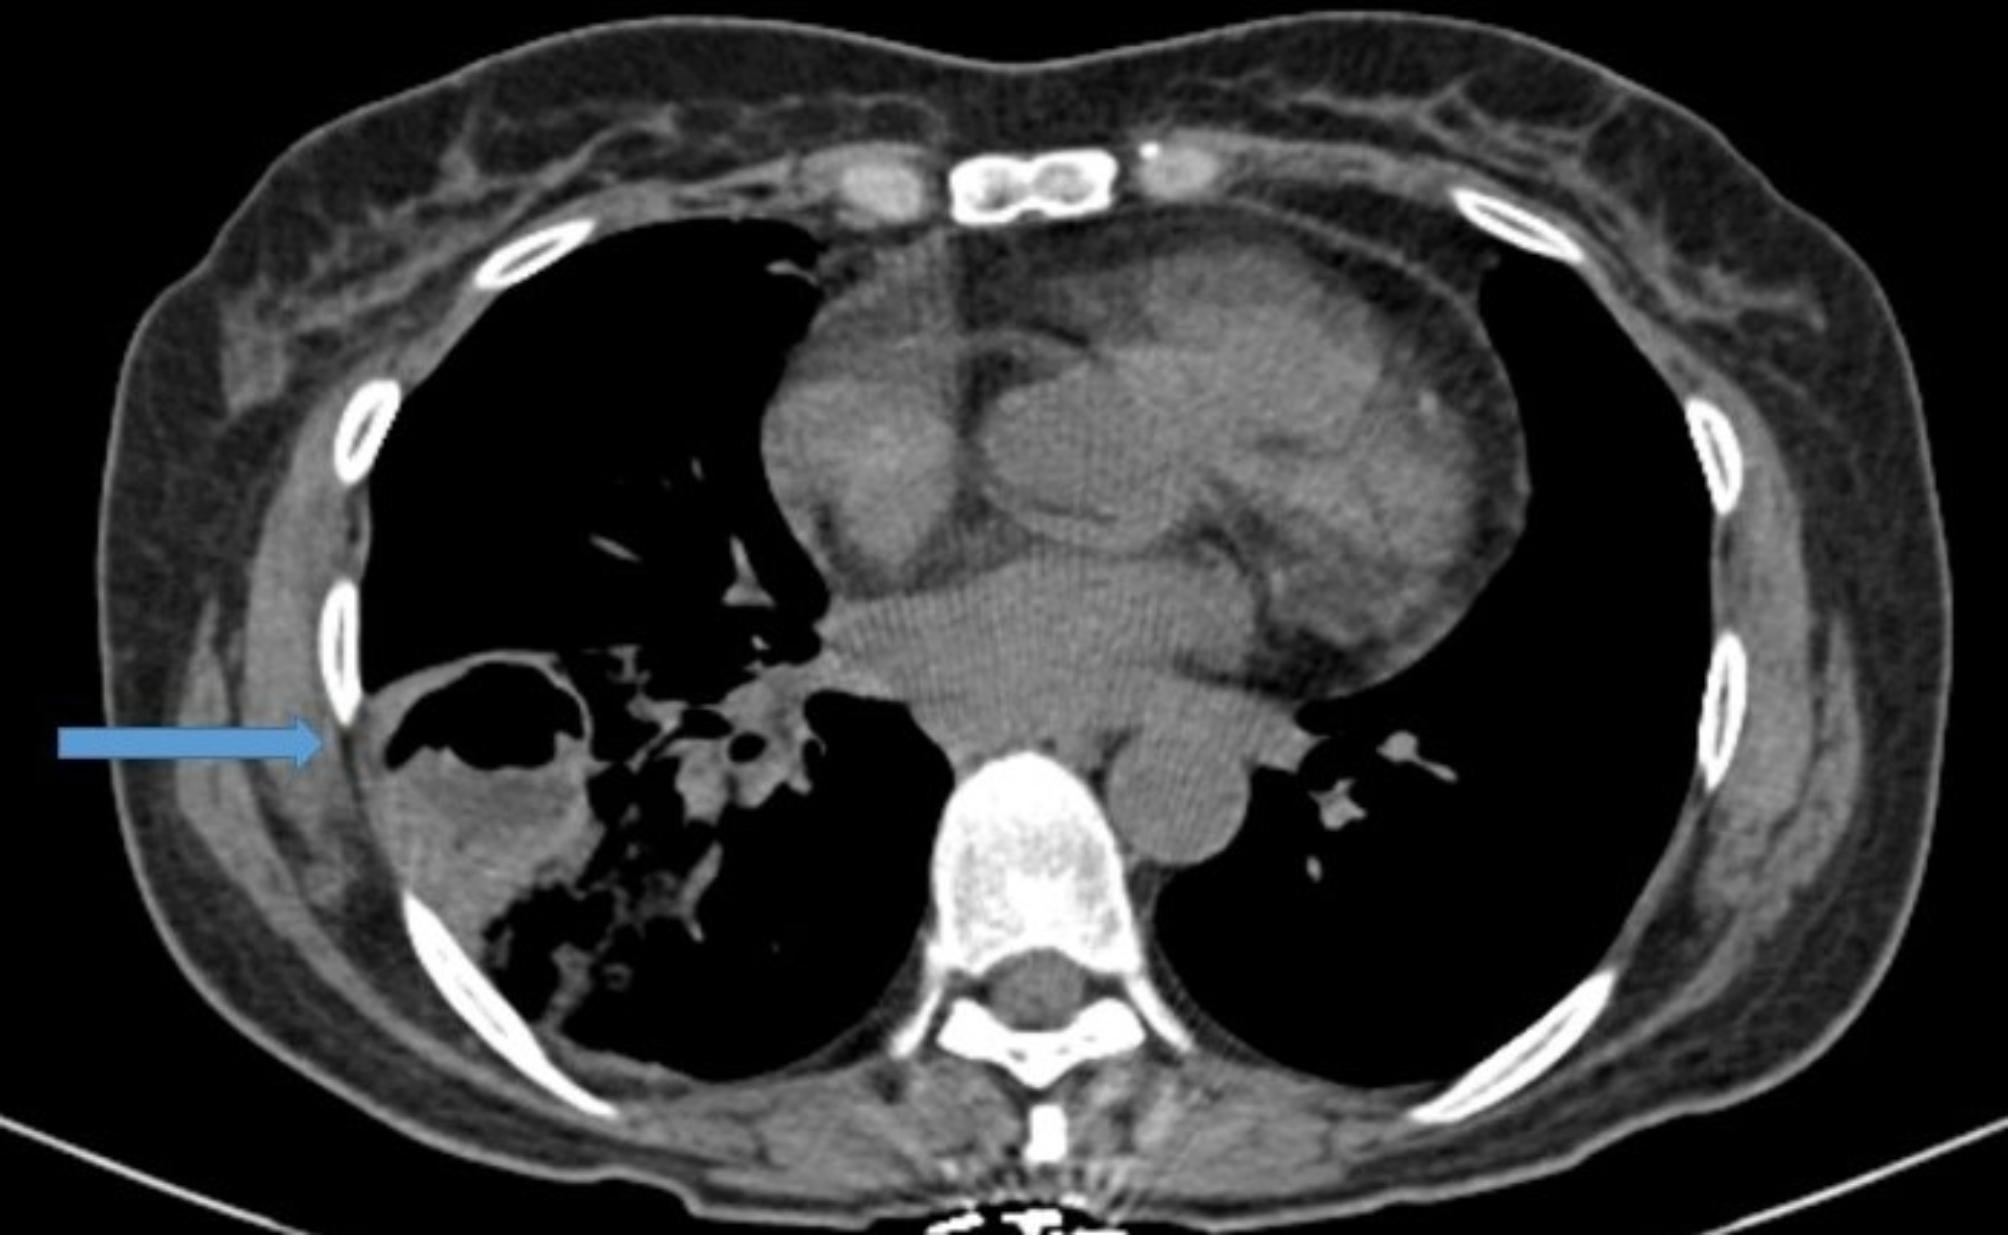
Mediastibal view of lung CT scan shows a cavity with thick, regular walls and undulating air-fluid levels measuring 43 × 30, evident in the upper segment of the RLL (blue arrow)
